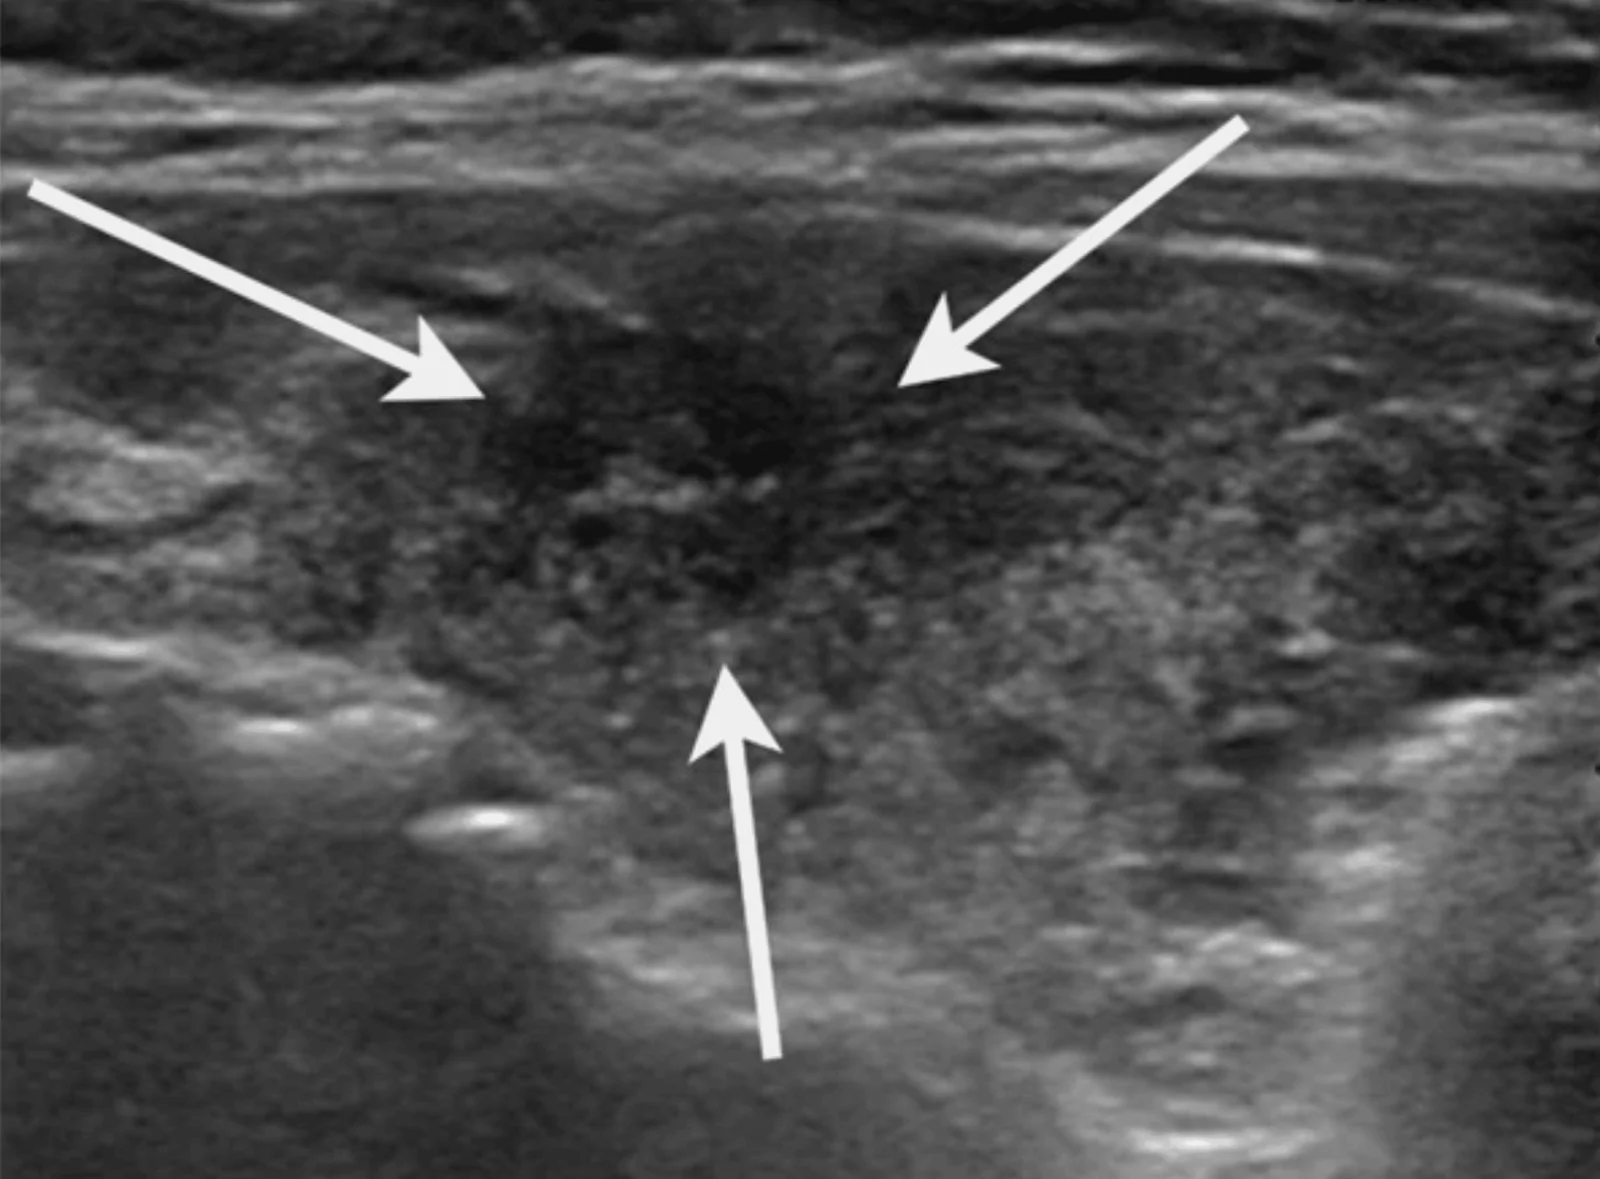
Heterogeneous thyroid with hypoechoic nodule. Longitudinal ultrasound image showing an irregular, hypoechoic nodule (white arrows) within a heterogeneous background of the thyroid lobe. Credit: Adapted from Park et al. [4], BMC Cancer. Used under the Creative Commons Attribution (CC BY) 2.0 International License, with cropping.
